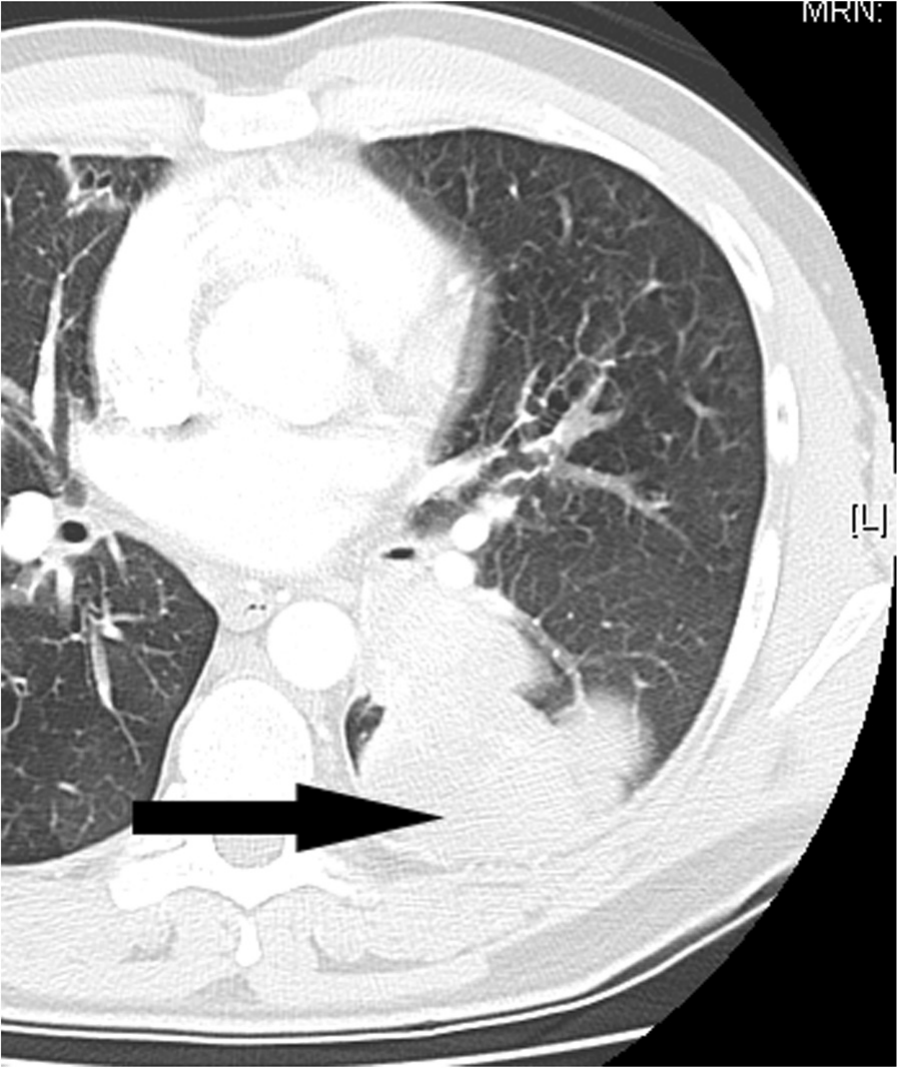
Computed tomography scan taken of a patient with a lung abscess that required surgical removal.*H*. *influenzae* was isolated from the lung tissue.
